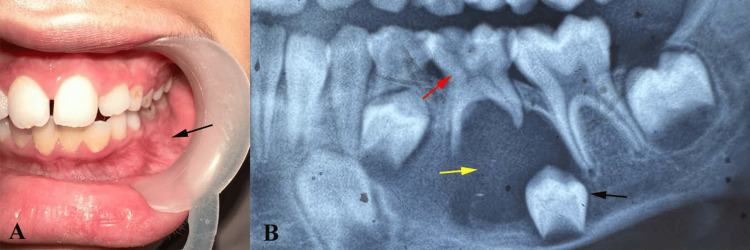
Intraoral exam and radiographic evaluation (A) Intraoral swelling in the posterior mandible obliterating buccal sulcus (black arrow) at the deciduous second molar. (B) Orthopantomogram showed a radiolucent cystic area of 2 x 3 cm in size (yellow arrow), a displaced tooth bud of the second premolar (black arrow), and an infected carious deciduous second molar (red arrow) Original images of the patient; used with the patient's permission
